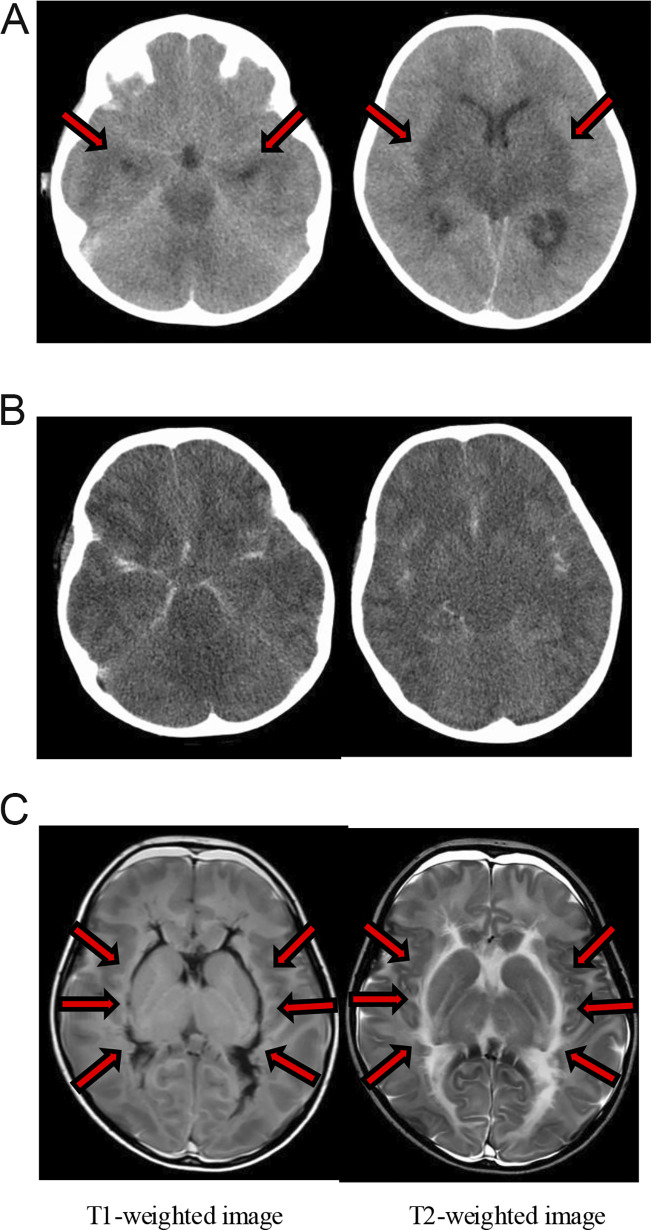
Brain CT and MRI of our patient. (A) Initial brain CT scan at disease onset showing hypoattenuation in the cerebral cortex, basal ganglia, thalamus, brainstem, and cerebellum (arrows), along with marked cerebral edema. (B) Follow-up brain CT on hospital day 28 demonstrating progression of hypoattenuation throughout the cerebral white matter. (C) Brain MRI on day 93 showing findings suggestive of liquefactive necrosis. T1-weighted image showed hypointensity, and T2-weighted image showed hyperintensity in the periventricular and external capsule regions (arrows), indicating parenchymal liquefactive necrosis.
